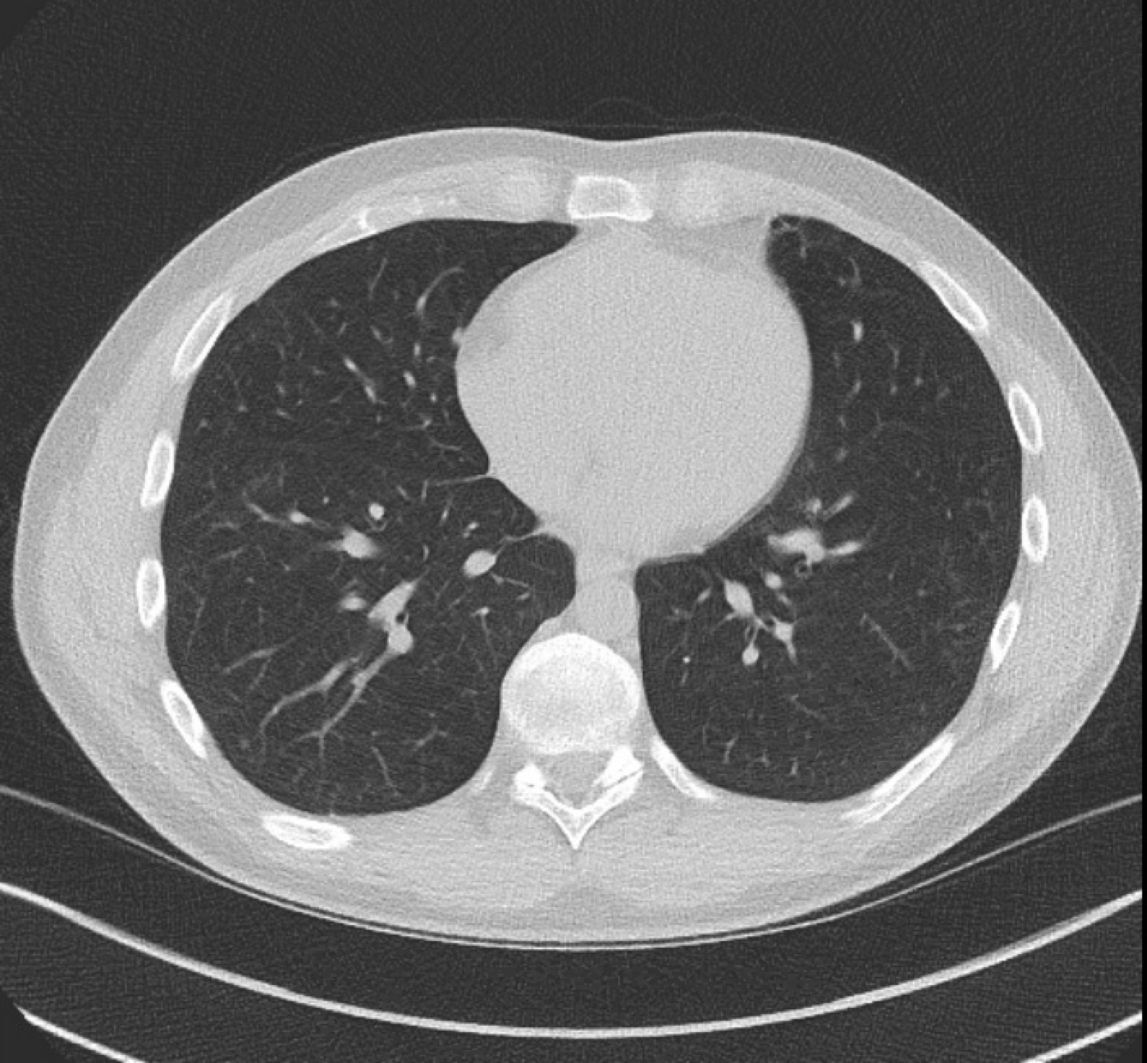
Follow-up computed tomography (CT) of the chest six weeks following discharge showing peribronchovascular ground-glass opacities/nodules in the right lower lobe that were likely due to resolving infection.
